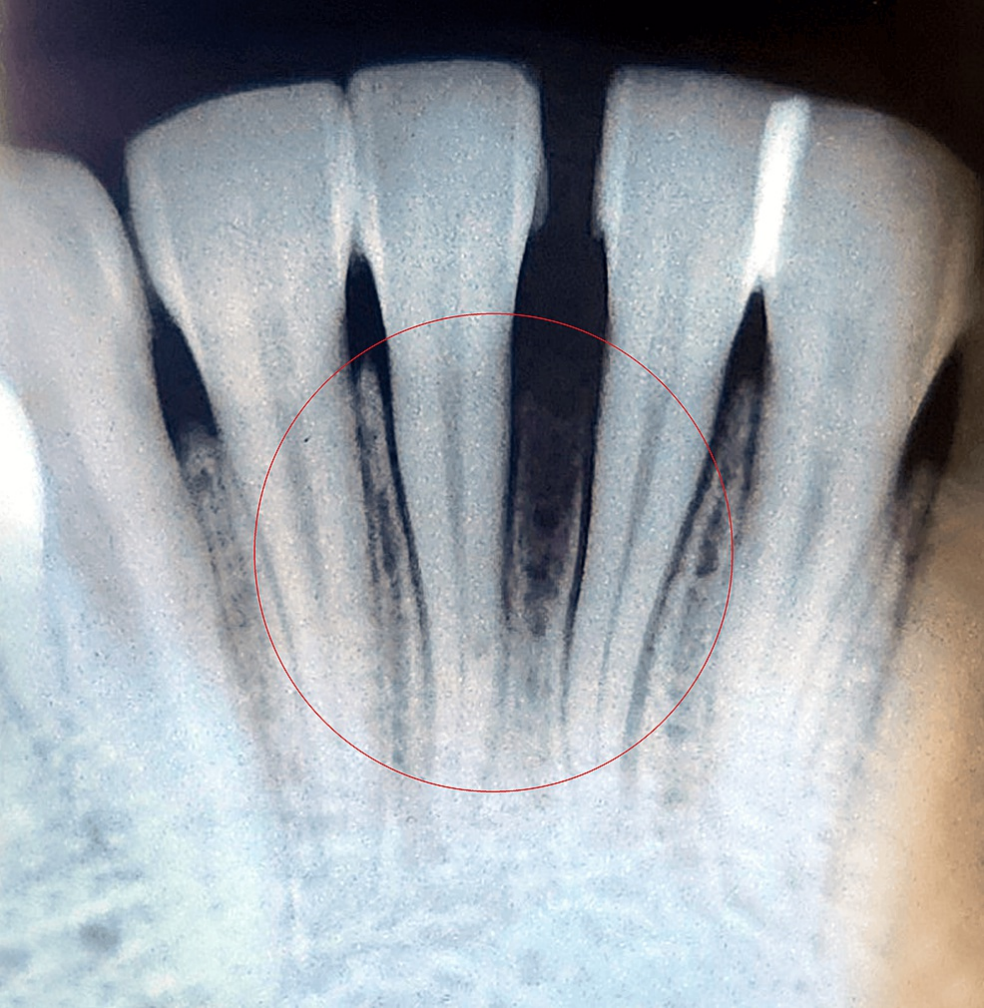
Intraoral periapical radiograph in 31, 41 region. Image Credit: Prasanna Sonar
